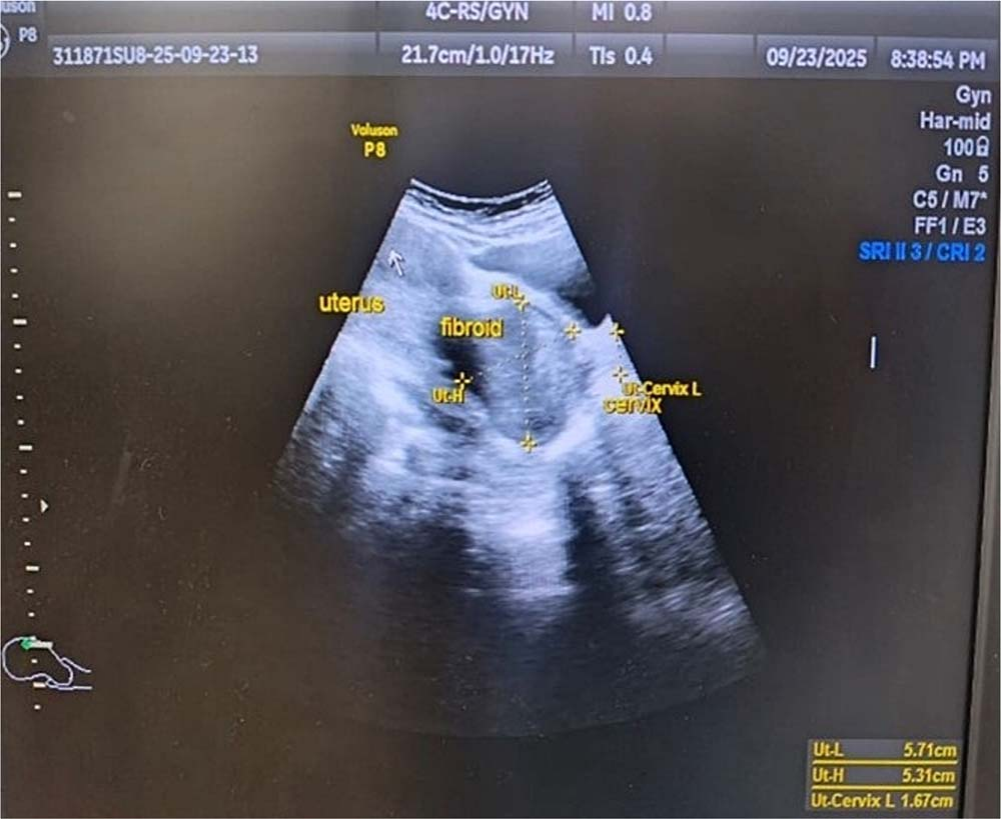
Ultrasonographic image of fibroid polyp of size 5.71 cm × 5.31 cm.
